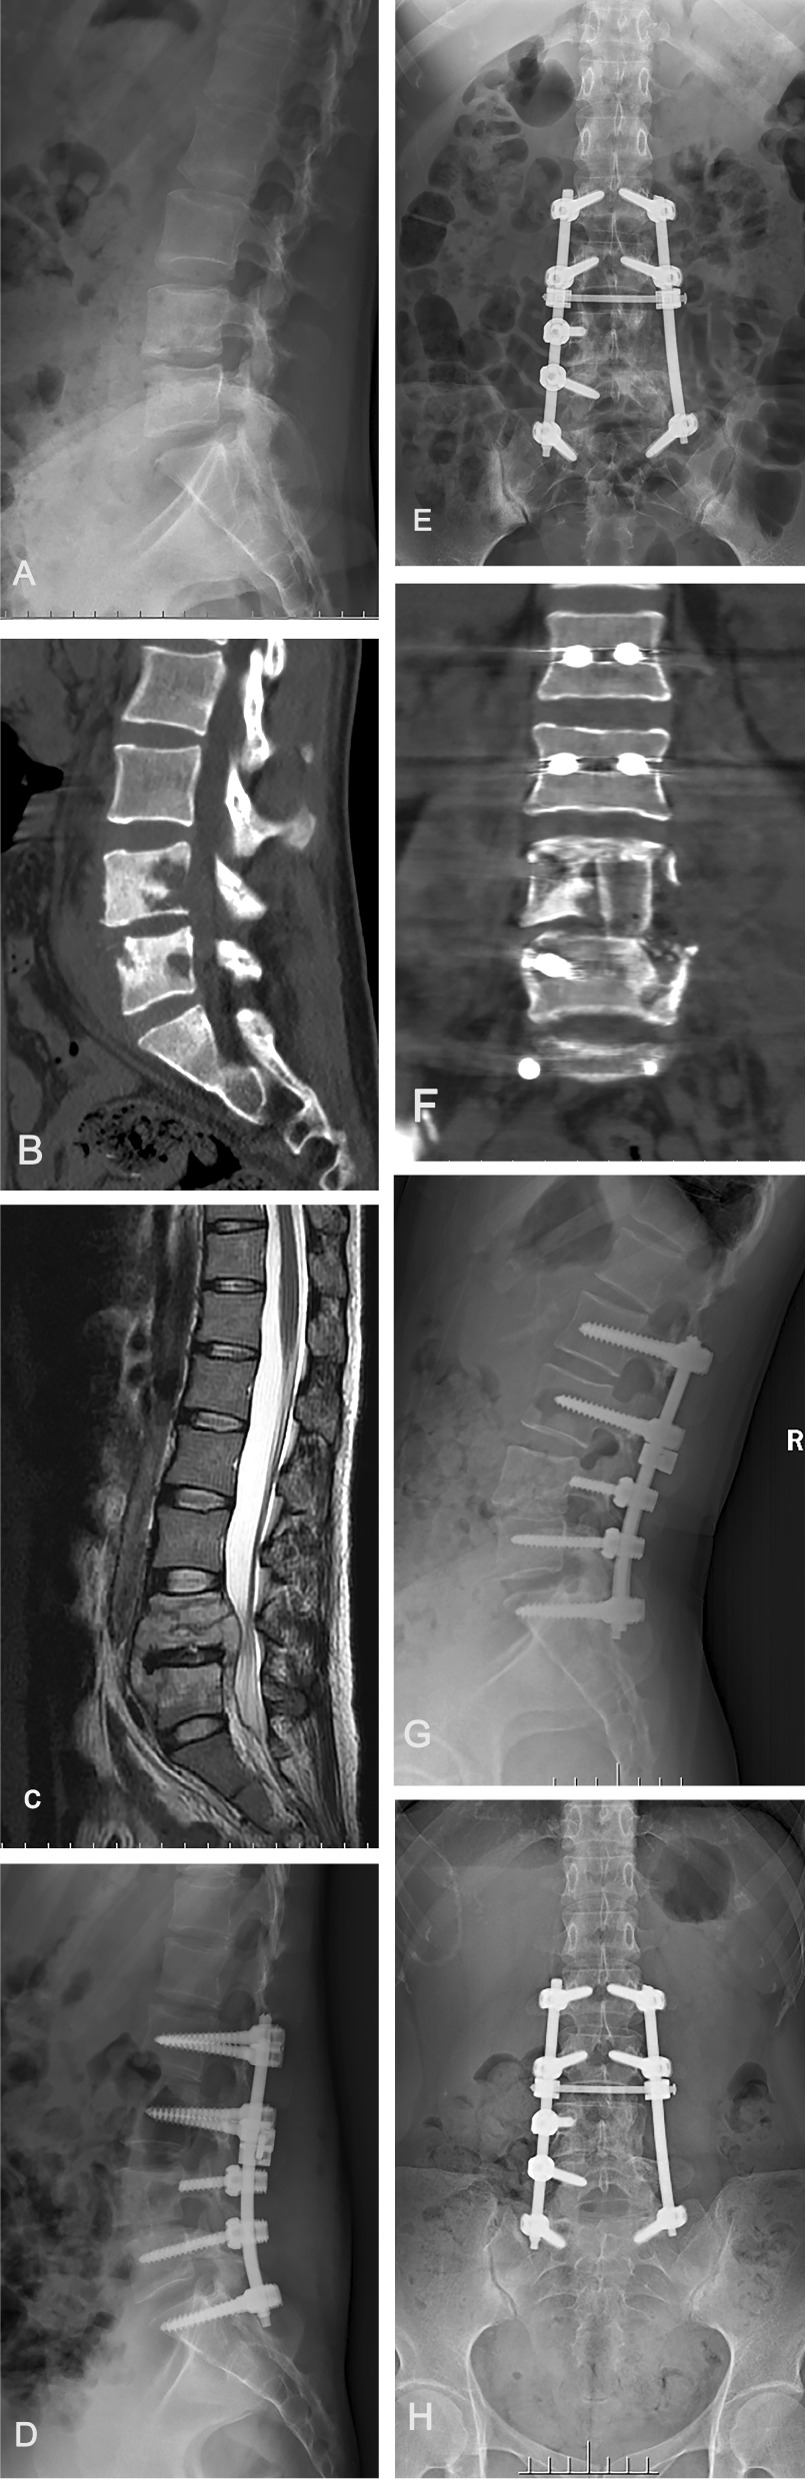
A 68-year-old male with L2–3 tuberculosis. a The lateral X-ray film before surgery demonstrated intervertebral stenosis between the L2–3. b, c Preoperative CT and MRI showed that L2–3 vertebral bone destruction. d–f Postoperative X-ray and CT of a patient who underwent posterior internal fixation from T12 to L5 and morselized bone graft at L2–3. g, h X-ray at 26 months postoperative showing bone fusion between L2 and L3, without signs of tuberculosis recurrence and hardware failure
